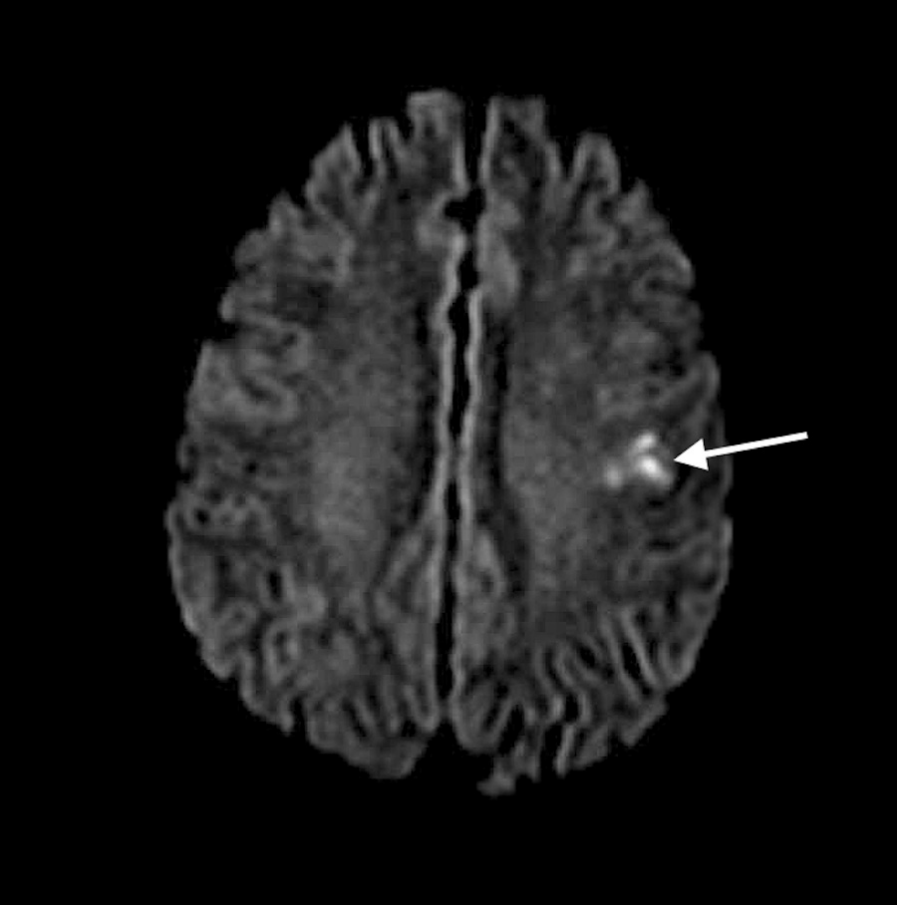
Magnetic resonance imaging (MRI) of the brain showing area of diffusion restriction (arrow) in the left parietal region
